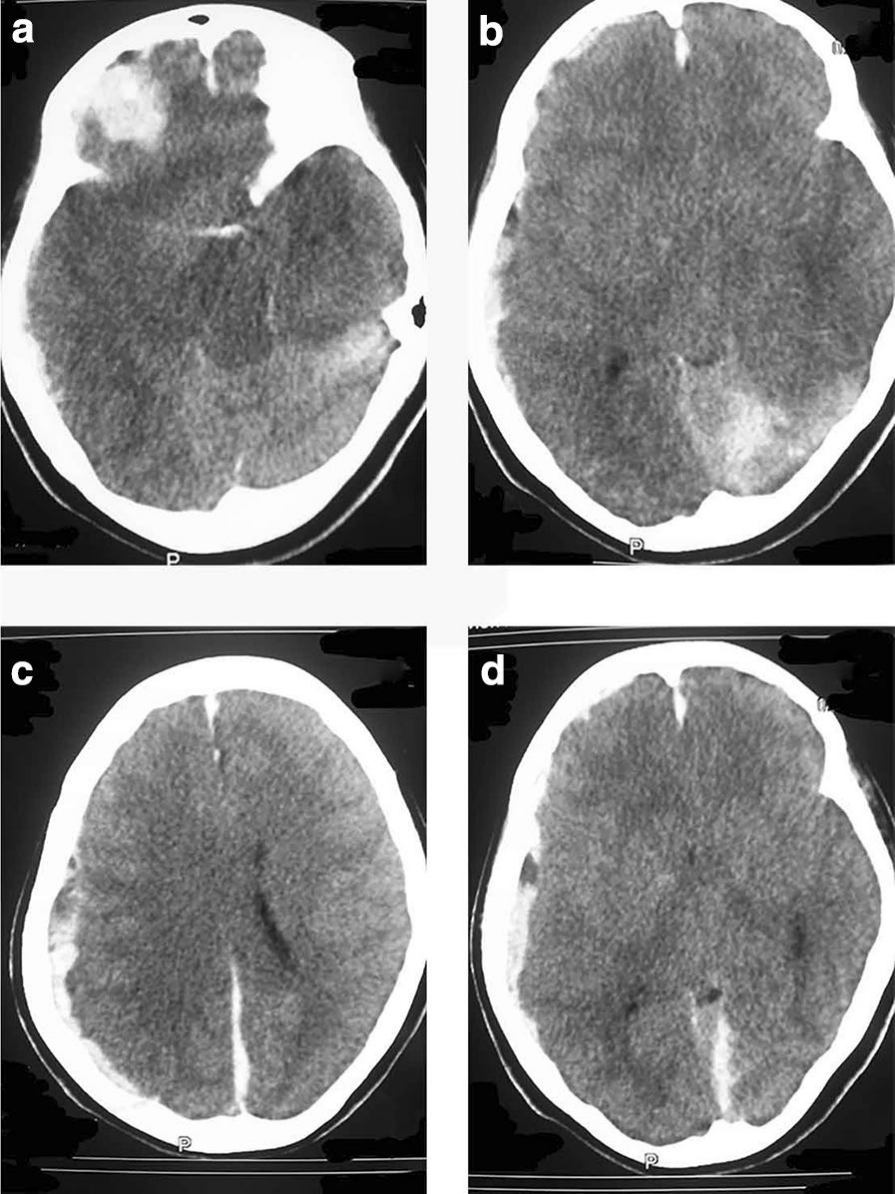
a, b Non contrast computer tomography (CT) brain showing *right* frontal, *left* parietal and occipital lobe subarachnoid hemorrhages and intra cranial hemorrhages. c, d Non contrast computer tomography (CT) brain showing *right* subdural hematoma
